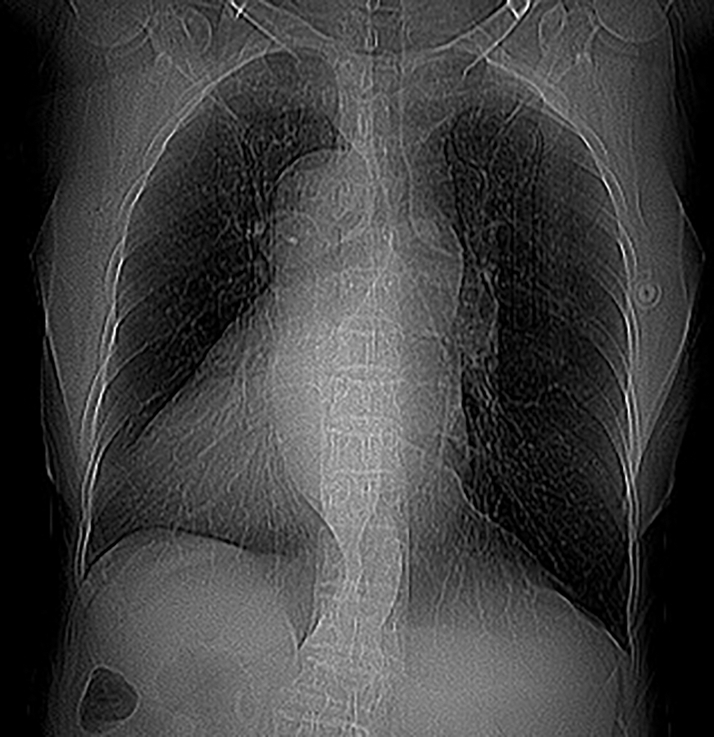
Chest X-Ray Demonstrating Mirror-Image Dextrocardia
